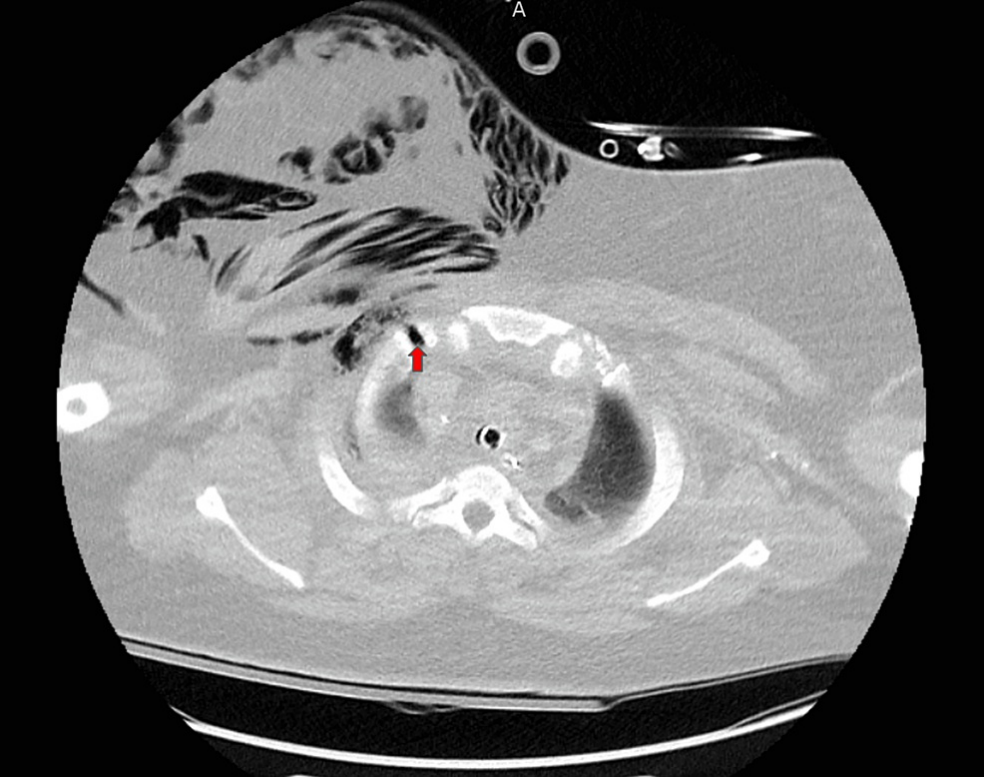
Axial CT with contrast shows the right-sided pleurocutaneous fistula (red arrow) with resulting subcutaneous emphysema of the right chest.
